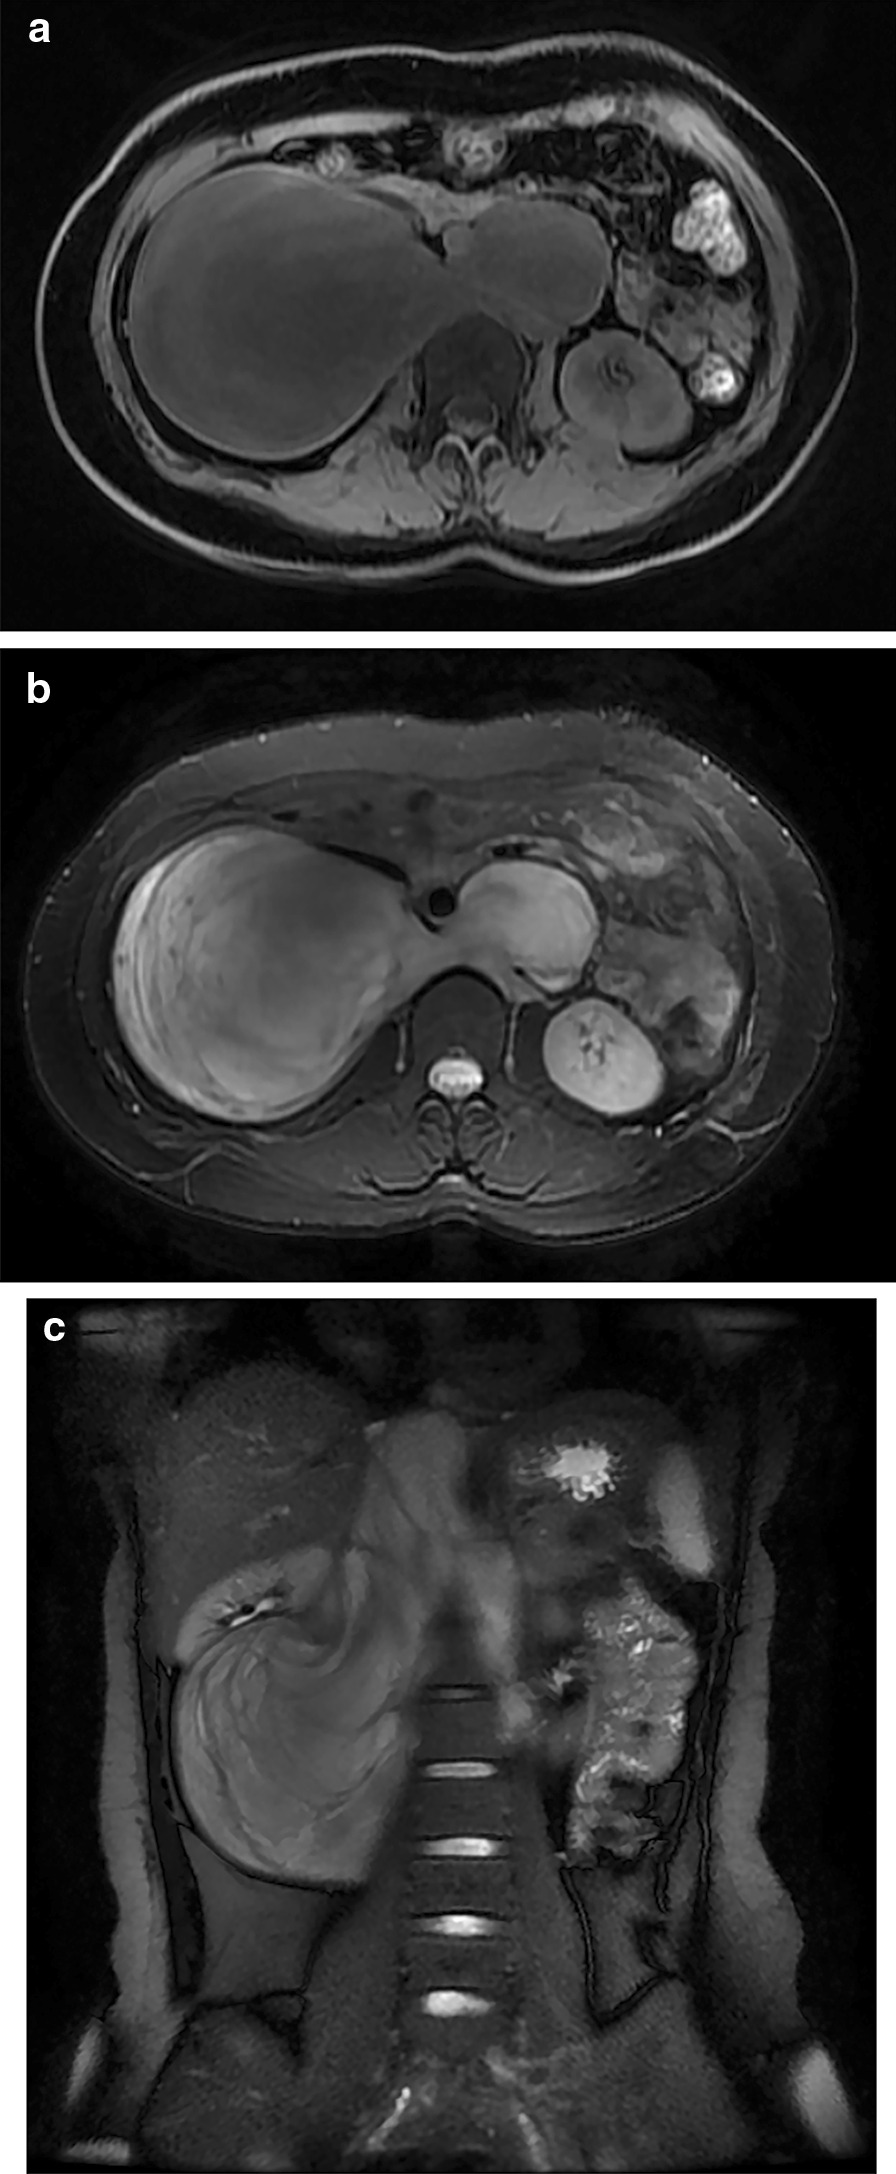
MR image of a 23-years-old female (patient no.25). The retroperitoneum ganglioneuroma was incidentally detected by a health check-up CT examination. a T1-weighted image. b axial T2-weighted image. c. coronal T2-weighted image. The whorled sign of retroperitoneum ganglioneuroma could be observed on T2-weighted image (b, c). The large amount of myxoid stroma presented predominantly high signal intensity on T2-weighted images and the cellular components including Schwann cells and ganglions may be responsible for the interlaced or nodular low signal intensity
